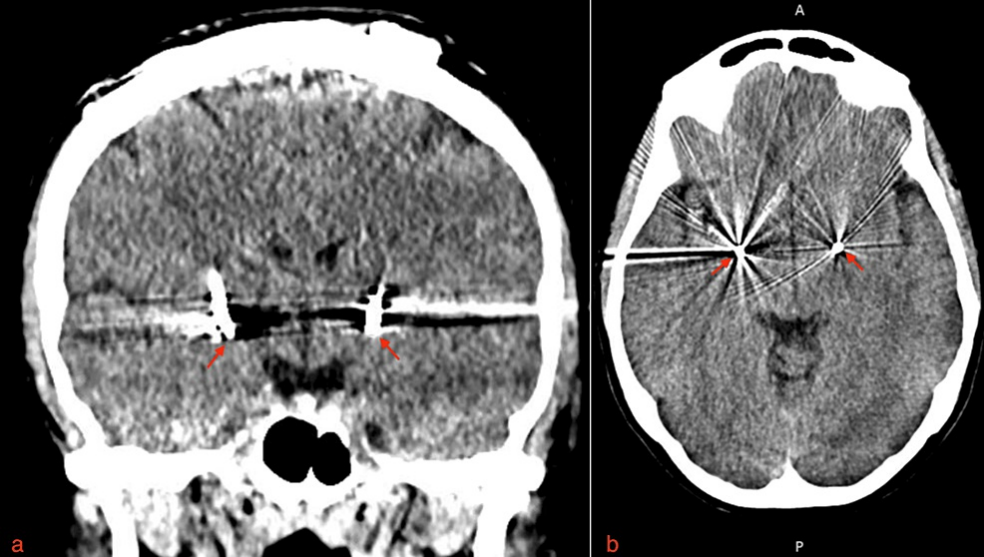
Coronal view (a) and axial view (b) of head CT depict bilateral deep brain stimulation electrodes placed in the globus pallidus internus
